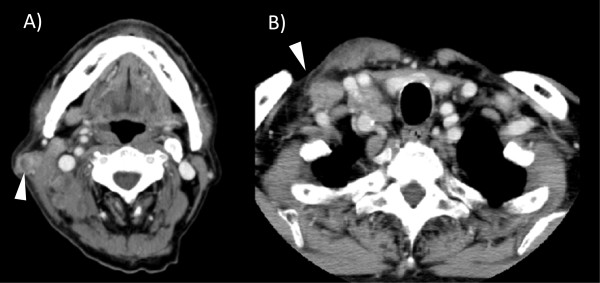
Contrast-enhanced computed tomography scan. Arrowheads point to multiple enlarged lymph nodes at the lateral margin of the right sternocleidomastoid muscle (A) and in the supraclavicular region (B).
